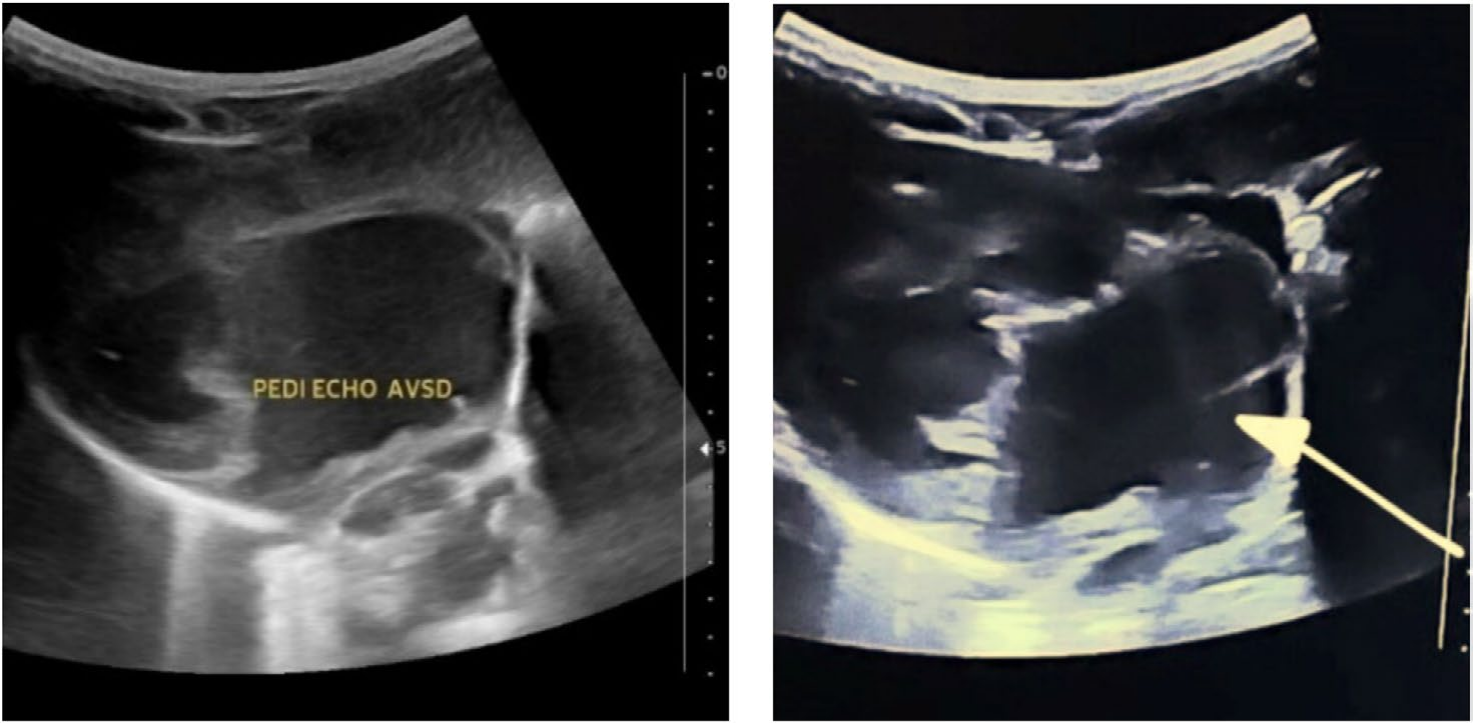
Echocardiography showing 1.5‐cm‐wide open atrioventricular septal defect involving both mitral and tricuspid valves (white arrow) insertion which shows large atrioventricular septal defect with dextrocardia and situs inversus.
